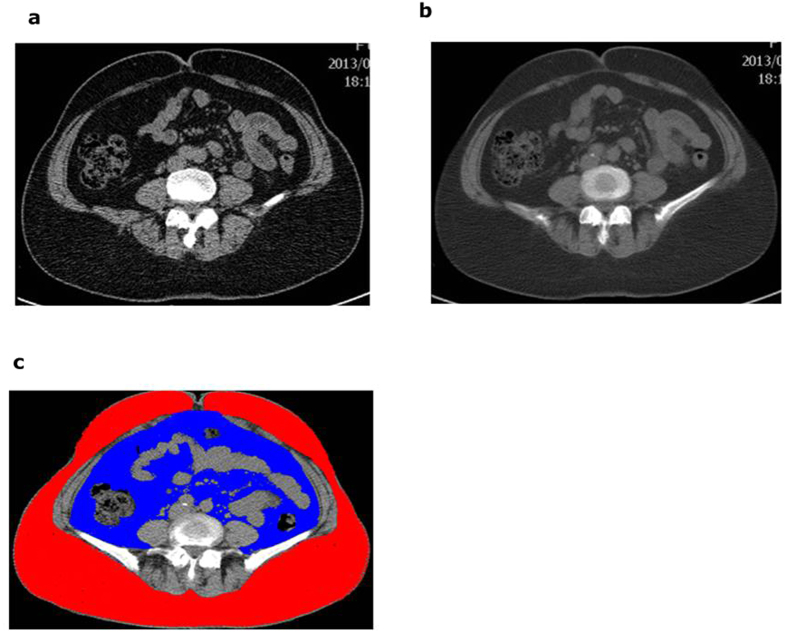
Axial views of an abdominal CT scan. An image taken through the umbilical plane with slice thickness of 2.5 mm. (b) A smooth image with the slice thickness of 10 mm was reconstructed from four 2.5-mm thick contiguous slices. (c) The SAT and VAT area estimated by AZE Virtual Place software from the reconstructed image (
